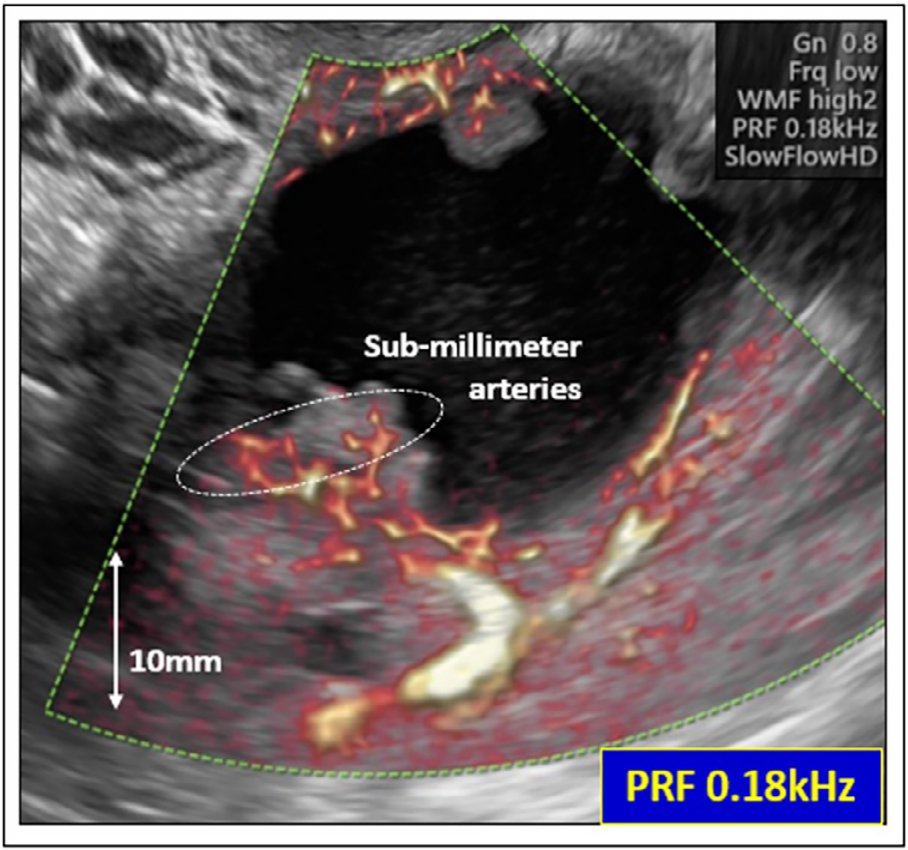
Example of microvascular imaging presenting extremely small, thin blood vessels of the size of arterioles in a borderline ovarian tumor using a 9–5 MHz transvaginal ultrasound transducer set to a pulse repetition frequency of 0.18 kHz.
